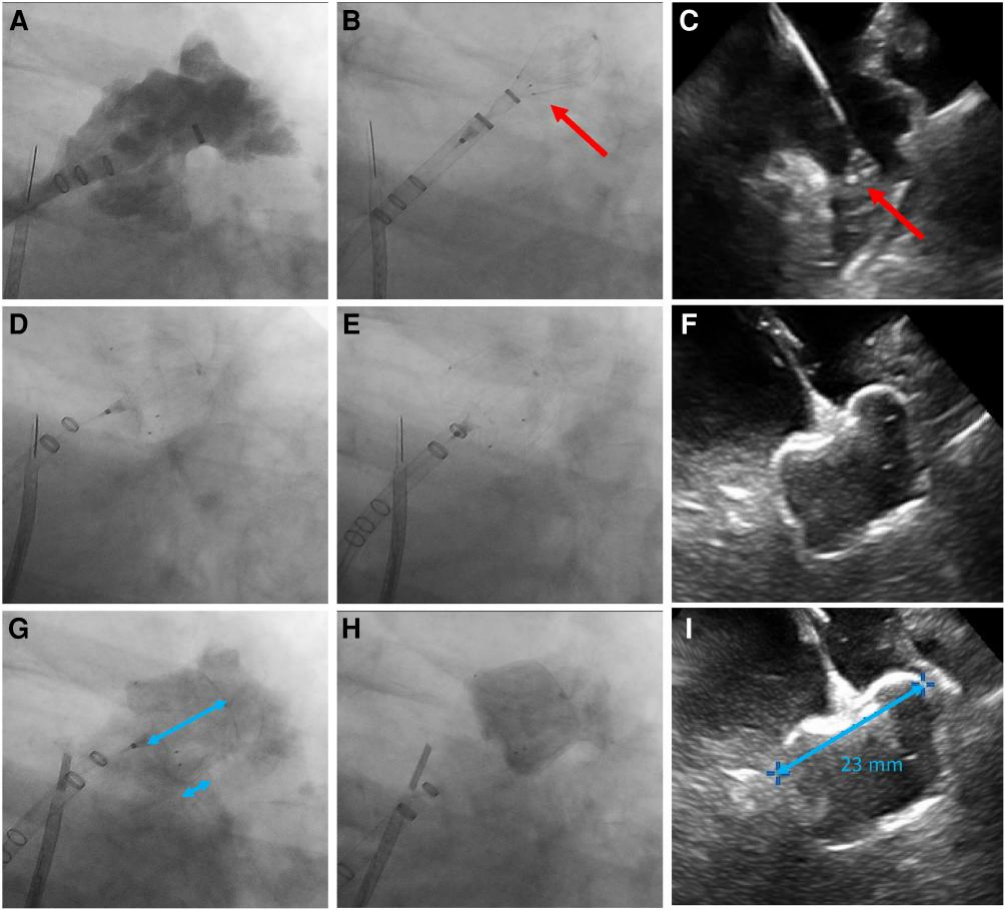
Fluoroscopic and intracardiac echocardiography images showing left atrial appendage closure with the WATCHMAN FLX Pro device. (*A*) Selective left atrial appendage angiogram. (*B* and *C*) Device in ball shape and arrow points to the three radiopaque markers. (*D*) Device fully deployed. (*E*) Tug test performed by pulling in the device with the delivery cable. (*F*) Intracardiac echocardiography image showing the fully deployed device. (*G*) Left atrial appendage angiogram with the three markers aligned in a straight line. The blue bars show the length of the small infero-posterior shoulder and the full length of the device. (*H*) Device released from the core wire. (*I*) Intracardiac echocardiography image showing a shoulder-to-shoulder measurement to estimate device compression.
